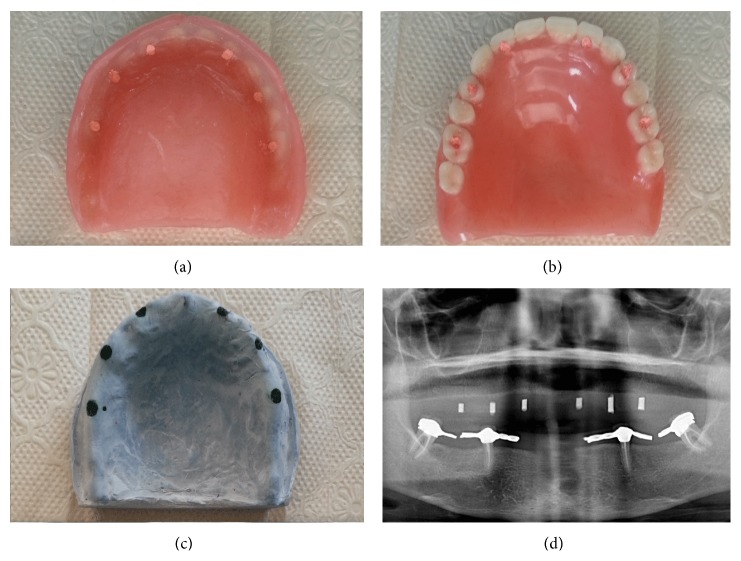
((a), (b)) The images of temporary denture with gutta percha filling material and (c) stone model that the estimated implant places were marked. (d) Orthopantomographic view of the case with gutta percha filled temporary denture.
